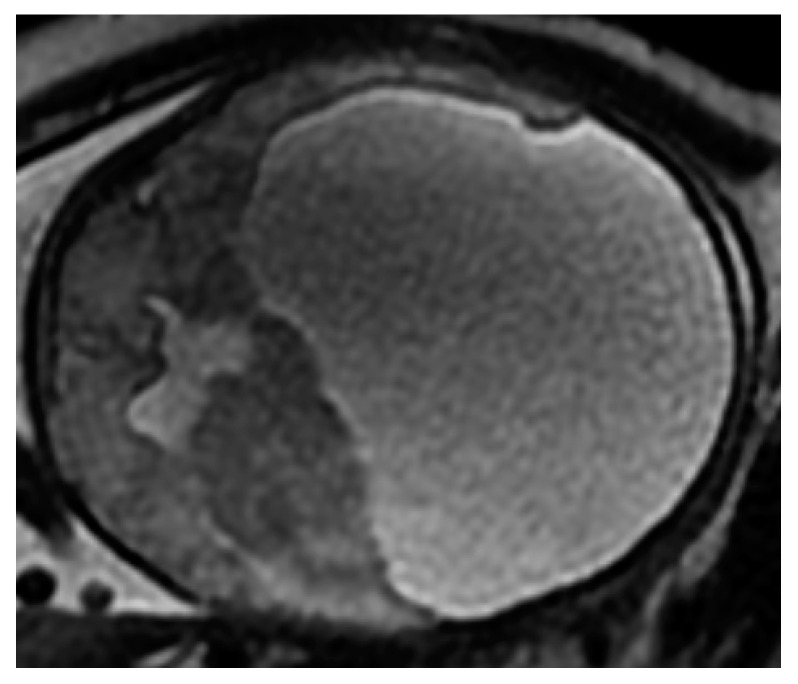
MRI picture of hydrancephaly.
